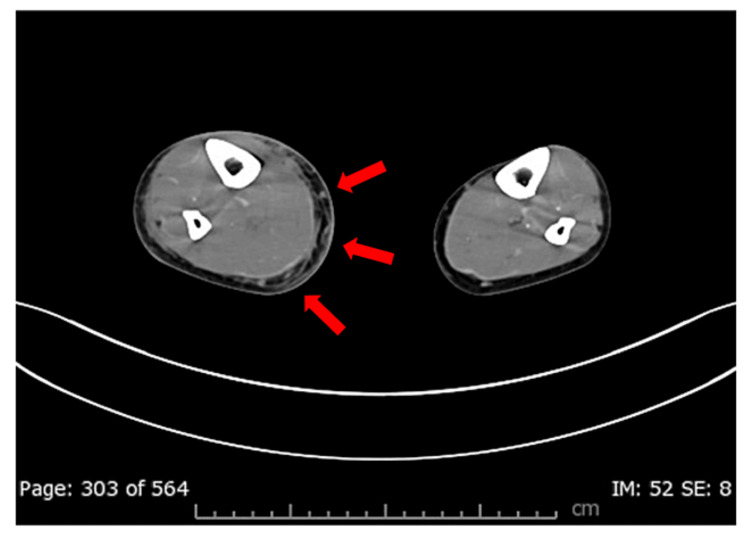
CT scan of the calves A CT scan of the calves showed evidence of swelling and subcutaneous edema in the right lower limb (red arrow).
